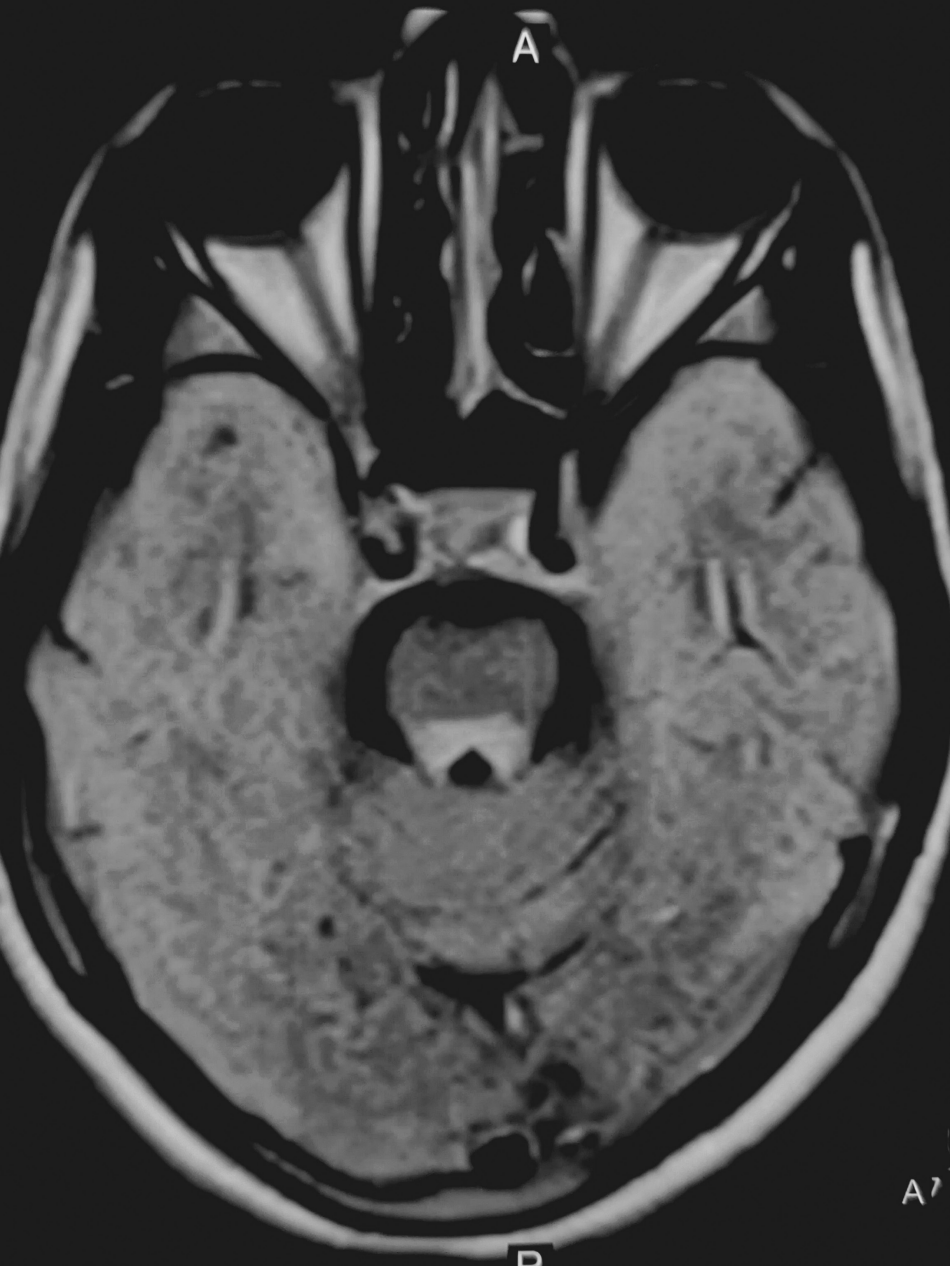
FLAIR hyperintensity in the dorsal pons.
